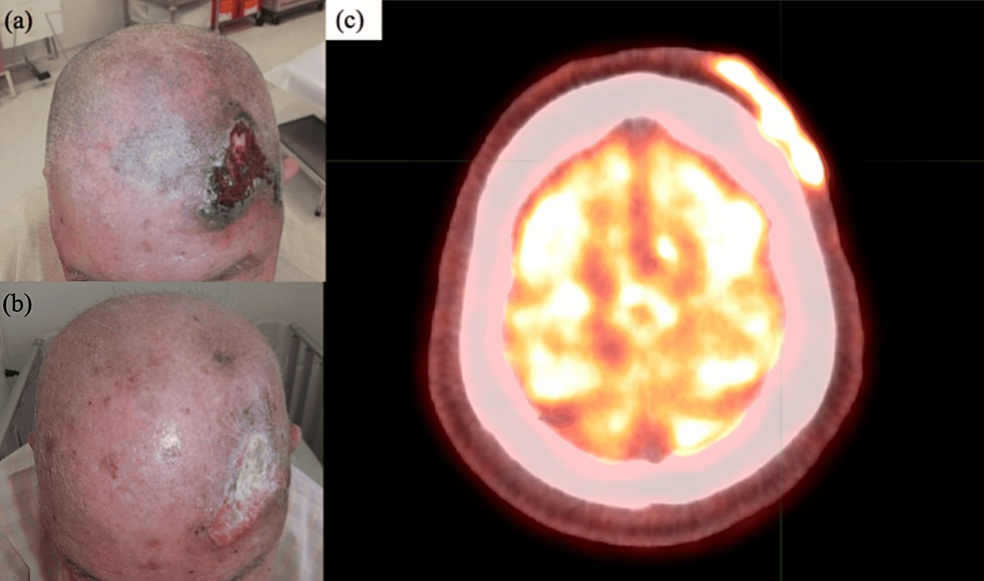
Case 2: (a) Scalp at initial examination; (b) Scalp during treatment (45Gy/15Fr); (c) Pre-treatment PET-CT. PET-CT: positron emission tomography-computed tomography
